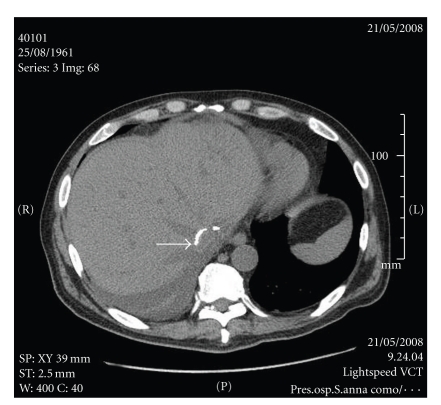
Postoperative CT scan (four months p.o), showing the liver graft with the patent Dacron prosthesis (arrow).
